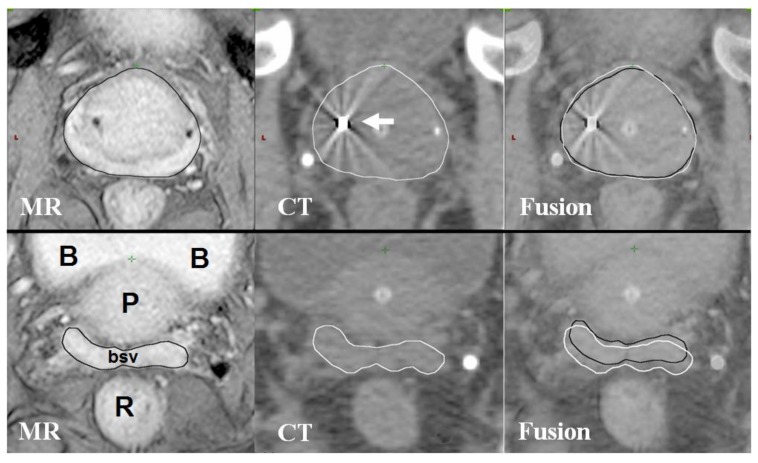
MR and CT fusion for prostate contouring.Axial MR and CT images at the level of the prostate (upper three images) and base of seminal vesicles (lower three images). B, bladder; P, prostate; bsv, base of seminal vesicles; R, rectum. White arrow shows gold fiducial marker on CT image. MRI-based contours are in black, CT-based contours are in white. The upper three images demonstrate good fusion of prostate MRI and CT imaging; lower three images from the same patient showing less accurate fusion at the base of seminal vesicles. The difference in position of bsv can be seen in the fusion images where contours from MRI (black) and CT (white) are shown overlapping.
